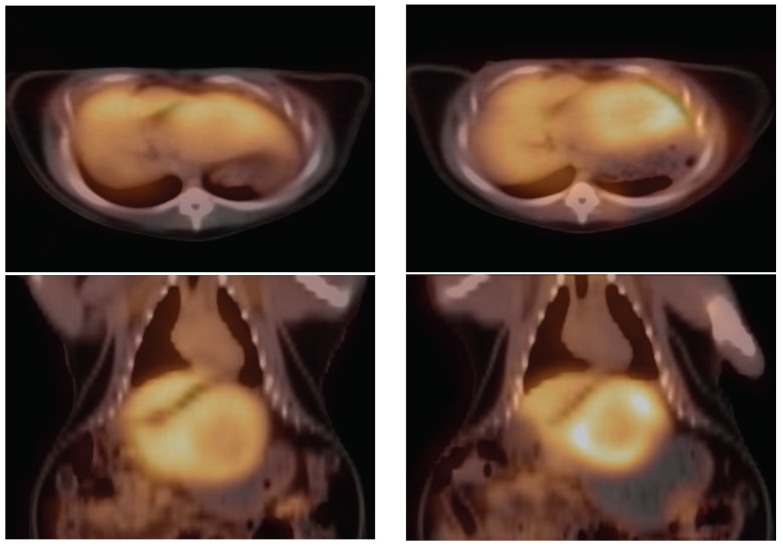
Comparing [^18^F]CFA scans. PET/CT overlays: Upper, trans-axial; lower, coronal. Right, baseline; left, application of cold CFA prior to [^18^F]CFA injection, with heightened uptake in the tumor rim comparing to the baseline.
